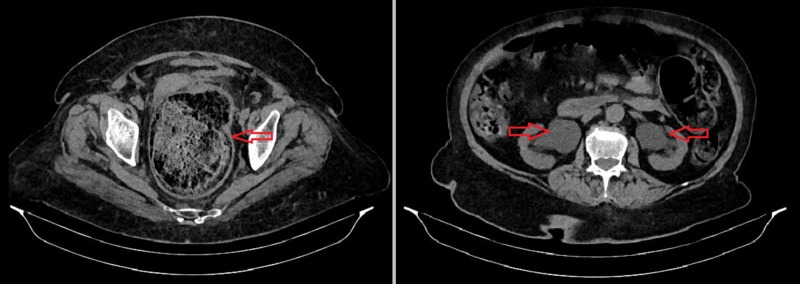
Non-contrast CT taken at admission Dilated and fecaloid-filled rectum and colon (left, red arrow) and bilateral hydroureteronephrosis in the urinary system (right, red arrows) can be seen CT: computed tomography
